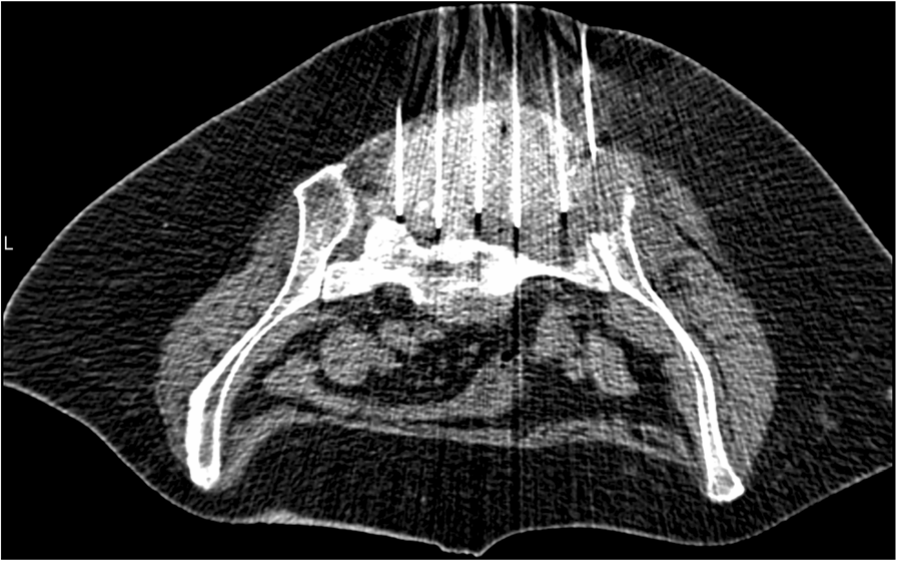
Intraprocedural computed tomography fluoroscopy image showing the six ablation electrodes in the tumor mass.
